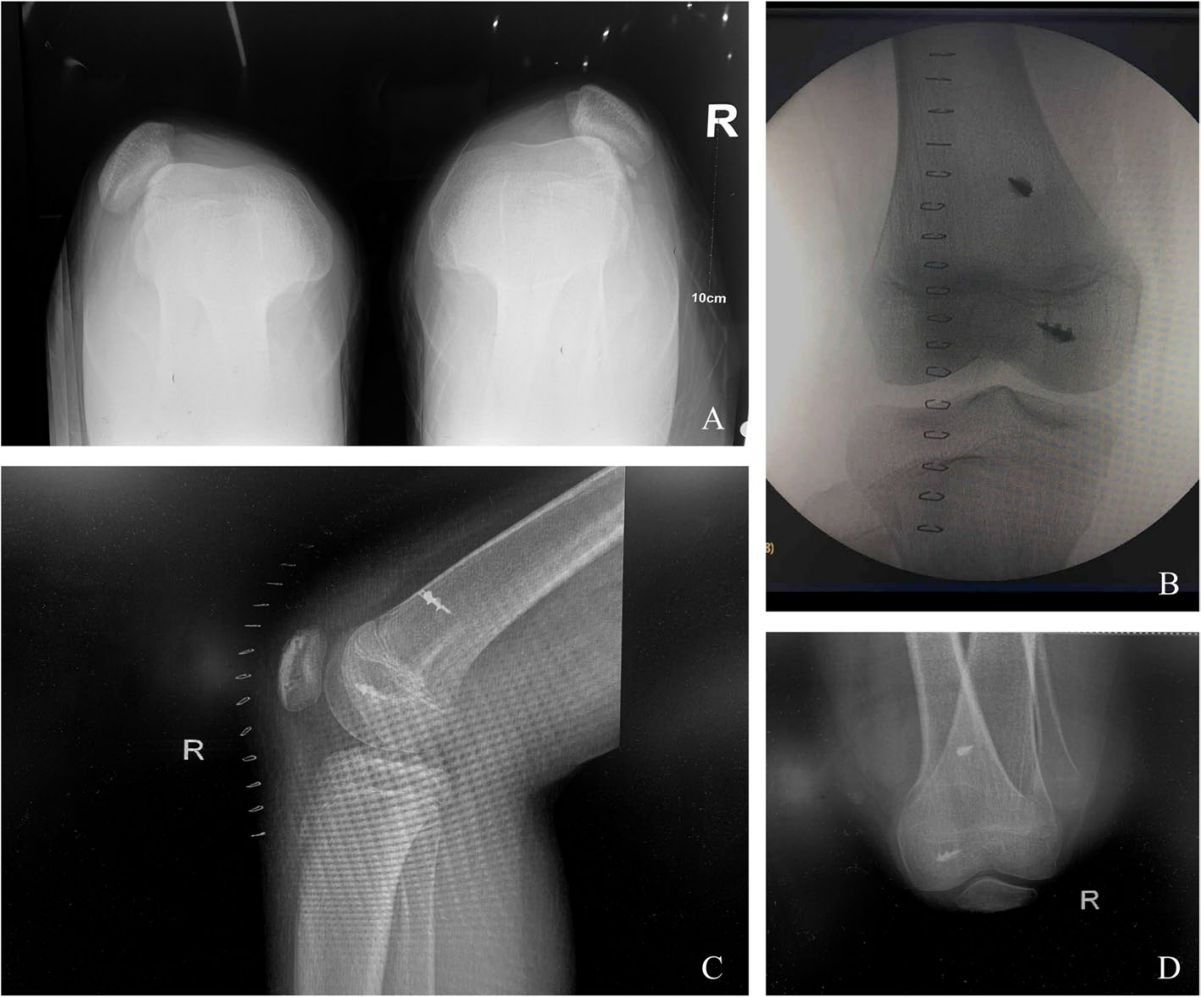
A–D. A: Preoperative computed tomograms of one patient. Postoperative anteroposterior (B), lateral (C), and axial (D) X-ray views of the knee joint
